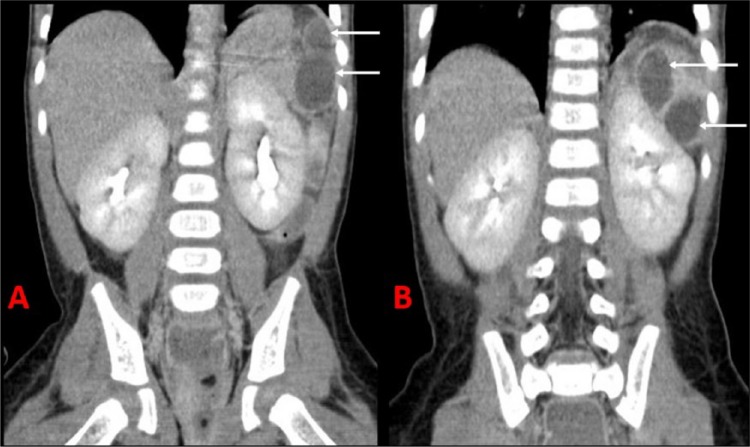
Computed tomography scan of the abdomen revealing multiple splenic abscesses (demonstrated by arrows) in the coronal views from hospital day 9 (A) and hospital day 41 (B).
